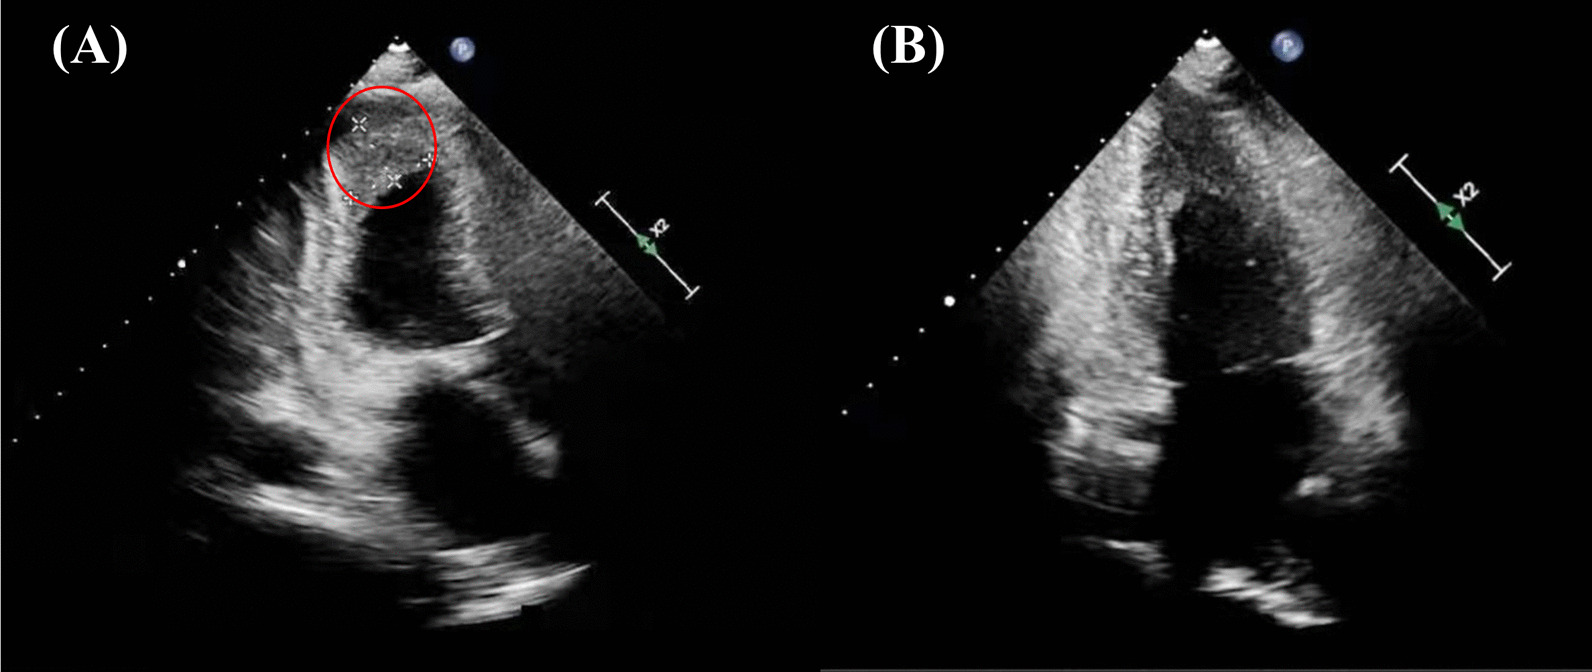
A Apical four-chamber view of the transthoracic echocardiogram showing thickened left ventricular endocardium and left ventricular thrombus formation (red circle). B Ten-month follow-up echocardiographic imaging after treatment showing thickening of the left ventricular apex and suspicion of apical thrombus
